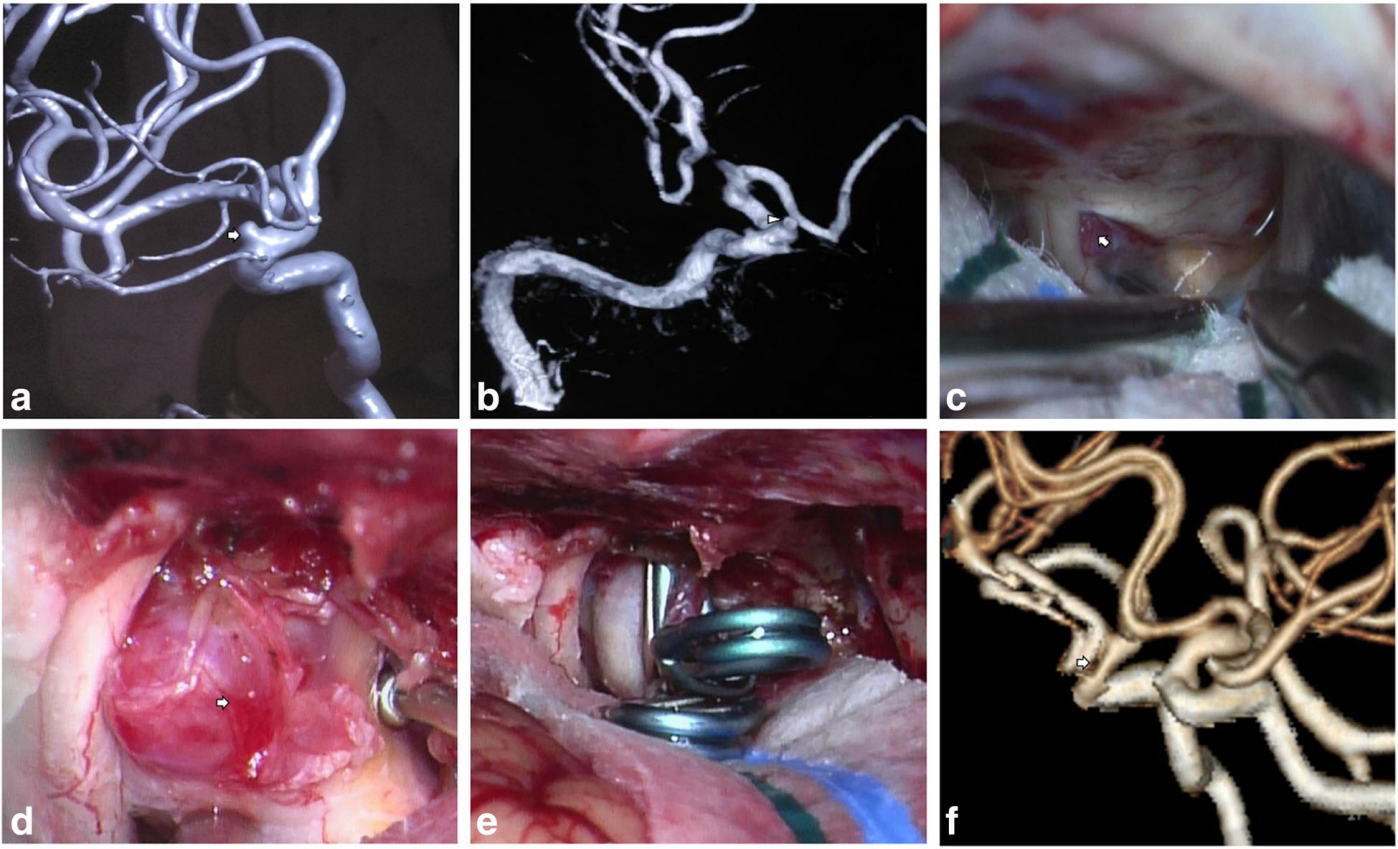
Case 14. a, b Preoperative 3D DSA demonstrated a hemispheric BLA (white arrow) and its growth accompanied with a daughter bleb (arrowhead). c This medially projecting BLA was barely visible underneath the optic nerve and the anterior clinoid process (white arrow). d The aneurysm and its translucent bleb (white arrow) originated from a partially sclerotic parent wall. e The aneurysm was obliterated. f Postoperative CTA showed 50% stenosis (white arrow) of the parent artery
